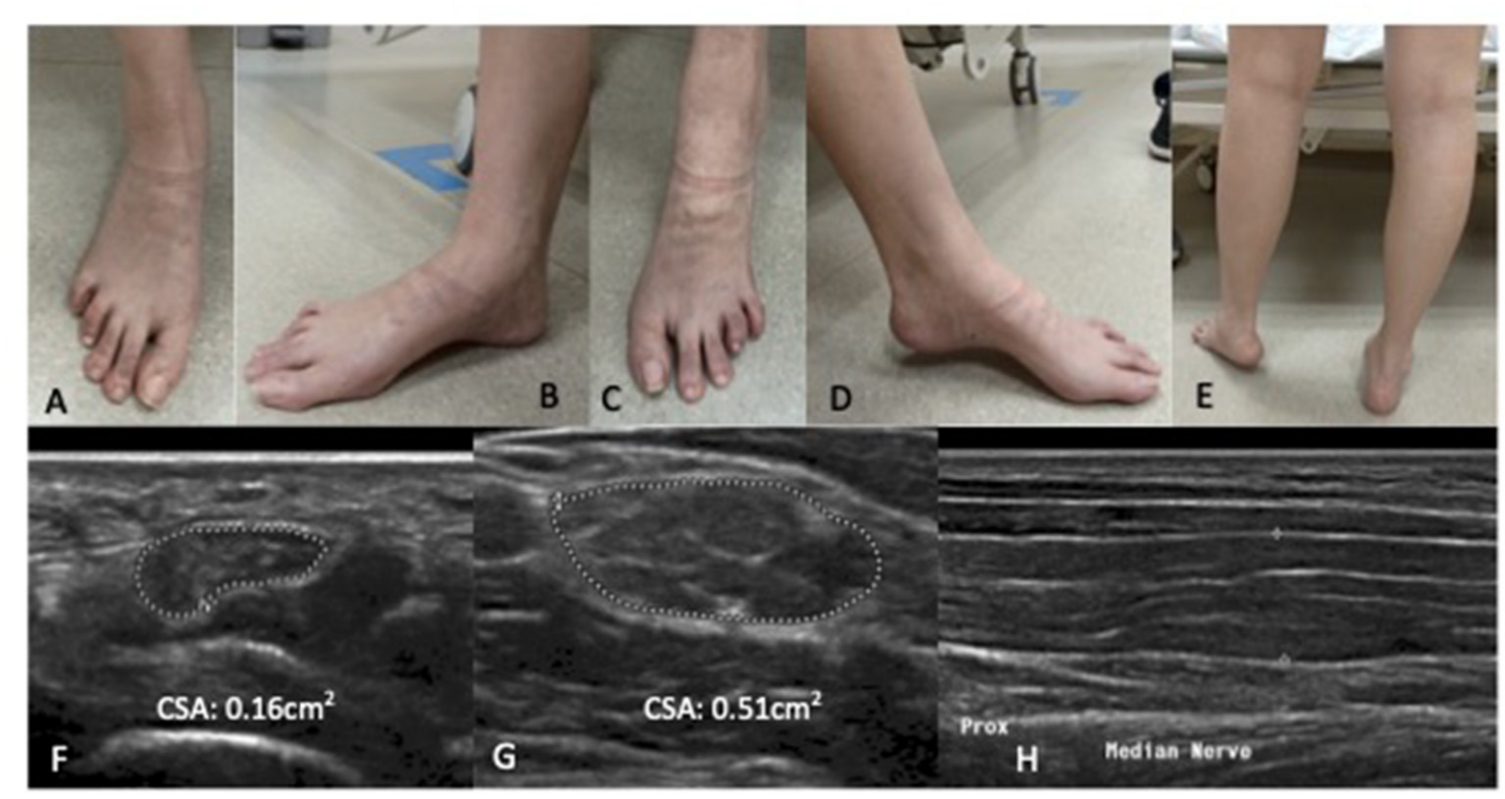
Patient one: Bilateral wasting of intrinsic foot muscles, mild elevation of bilateral plantar arches with claw toes. Left foot drop secondary to left common peroneal neuropathy at the fibular head. There is no significant calf wasting (E). (A,B) right foot; (C,D) left foot. High resolution ultrasound of the right median nerve findings at the carpal tunnel (F), at the elbow (G) showing marked diffuse increase cross sectional area more than 3× upper amit normal at non- entrapment sites with less marked enlargement at entrapment sites (H). (F,G) Transverse section; (H) Longitudinal section.
